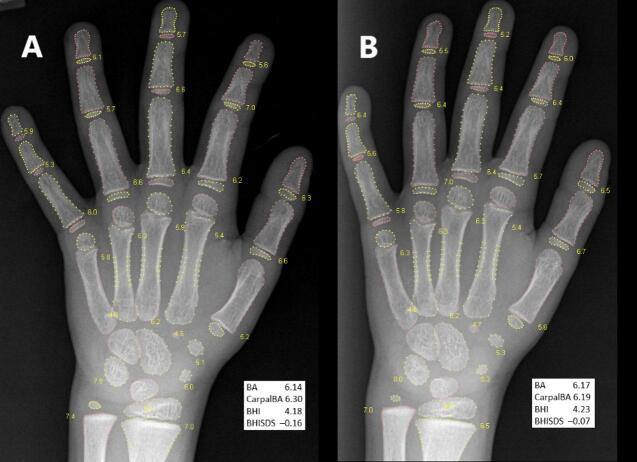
Posterior-anterior X-rays of the left (A) and right (B) hands of one participant obtained at the same visit. The right hand is shown mirrored. BoneXpert localizes the bone borders as indicated by the dotted lines, which are drawn in alternating yellow and red colors to aid the eye. The BoneXpert Greulich–Pyle bone ages of the individual bones are indicated next to the 21 tubular and 7 carpal bones. In the white boxes, the software has calculated the overall average bone age from the 21 tubular bones (BA) (standard Greulich–Pyle method) as well as an average from the carpal bones (carpal BA). In the shafts of the three middle metacarpals, BoneXpert’s localization of the cortex is indicated, which is used to compute the bone health index metric (BHI), and its Standard Deviations score (BHI SDS).
